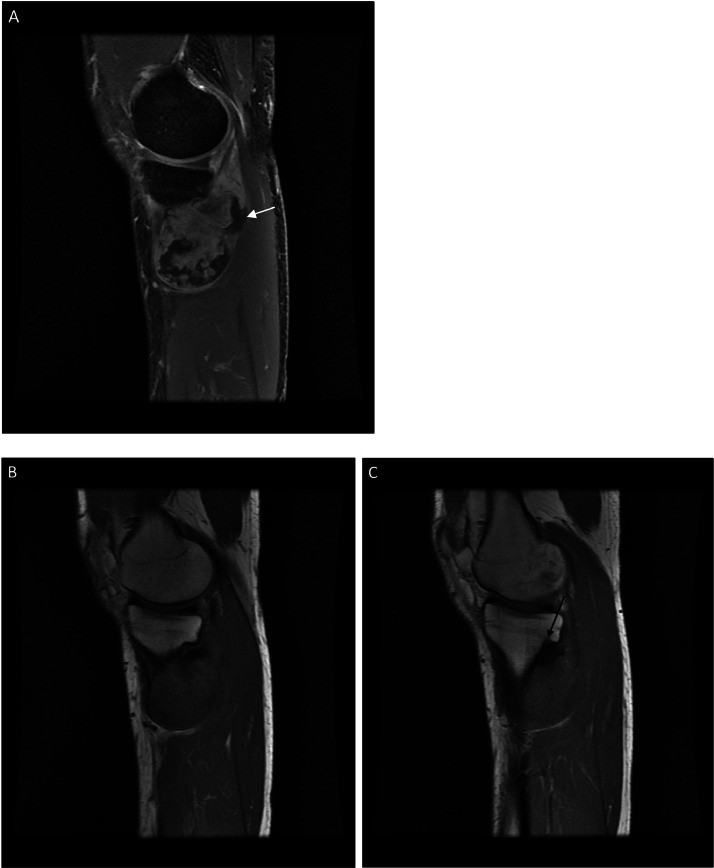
MRI of the left knee. (A) Sagittal fat-saturated T1-weighted image post intravenous contrast shows a heterogeneously enhancing mass arising from the cortex of the posteromedial tibial diaphysis with areas of nonenhancement along its periphery (white arrow). (B and C) Sequential sagittal T1-weighted images demonstrate the low T1 signal of the mass and medullary invasion at its superior portion (black arrow).
